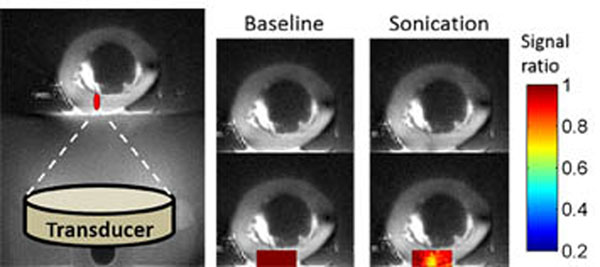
T1-weighted UTE images of a beef long bone during focused ultrasound application, showing the potential for MR thermometry using UTE MRI. To make the technique quantitative, the size of the T1-dependent signal decrease must be calibrated to temperature.
